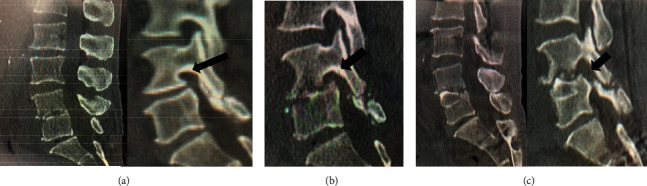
A 77-year-old male had severe back pain and could not sit for a long time due to pyogenic spondylodiscitis at the L4/L5 level. He underwent surgery with FED; however, not only did the postoperative pain scale scores remain unchanged, but also left thigh pain and quadriceps muscle weakness also appeared. He underwent anterior spinal fusion with an iliac strut autograft 13 days after FED. After the revision surgery, low back pain and neurological disorder improved. (a) Preoperative sagittal CT scan images showing destructive changes, including narrowing of disc height and destruction of the endplate at the L4/L5 level, although the foraminal sagittal view did not show foraminal stenosis (arrow). (b) Foraminal sagittal CT scan view after FED showing the progress of vertebral destruction and stenosis of the foramen (arrow). (c) Sagittal CT scan images showing regained foraminal space (arrow) after open surgery with anterior strut autograft.
